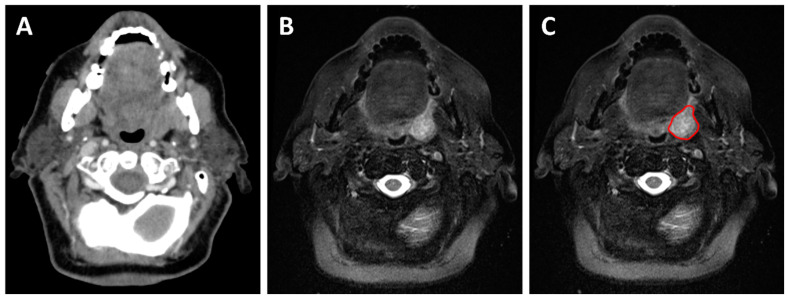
A 73-year-old patient with a cT2N1 p16-positive squamous cell carcinoma of the left tonsil planned for concurrent chemoradiotherapy. The planning contrast-enhanced CT (A) shows an asymmetrical left tonsil with poorly defined margins. The planning T2-weighted MRI (B,C) allows better visualization of the tumor margin and aids in the delineation of the gross tumor volume during radiation treatment planning (red contour).
